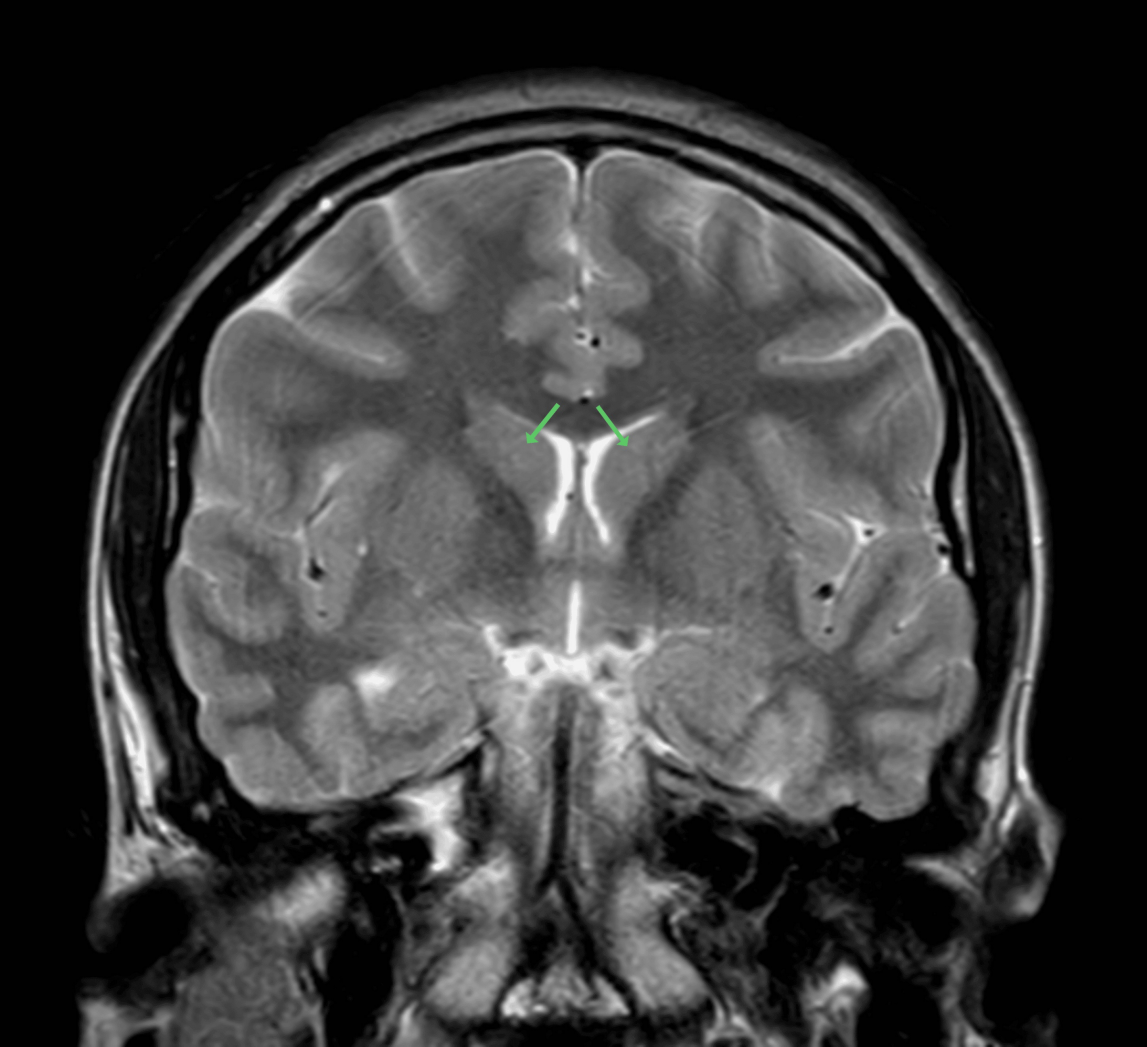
Coronal MRI MRI T2 image indicating enlarged caudate nuclei bilaterally (arrows) as a result of Sydenham's chorea.
